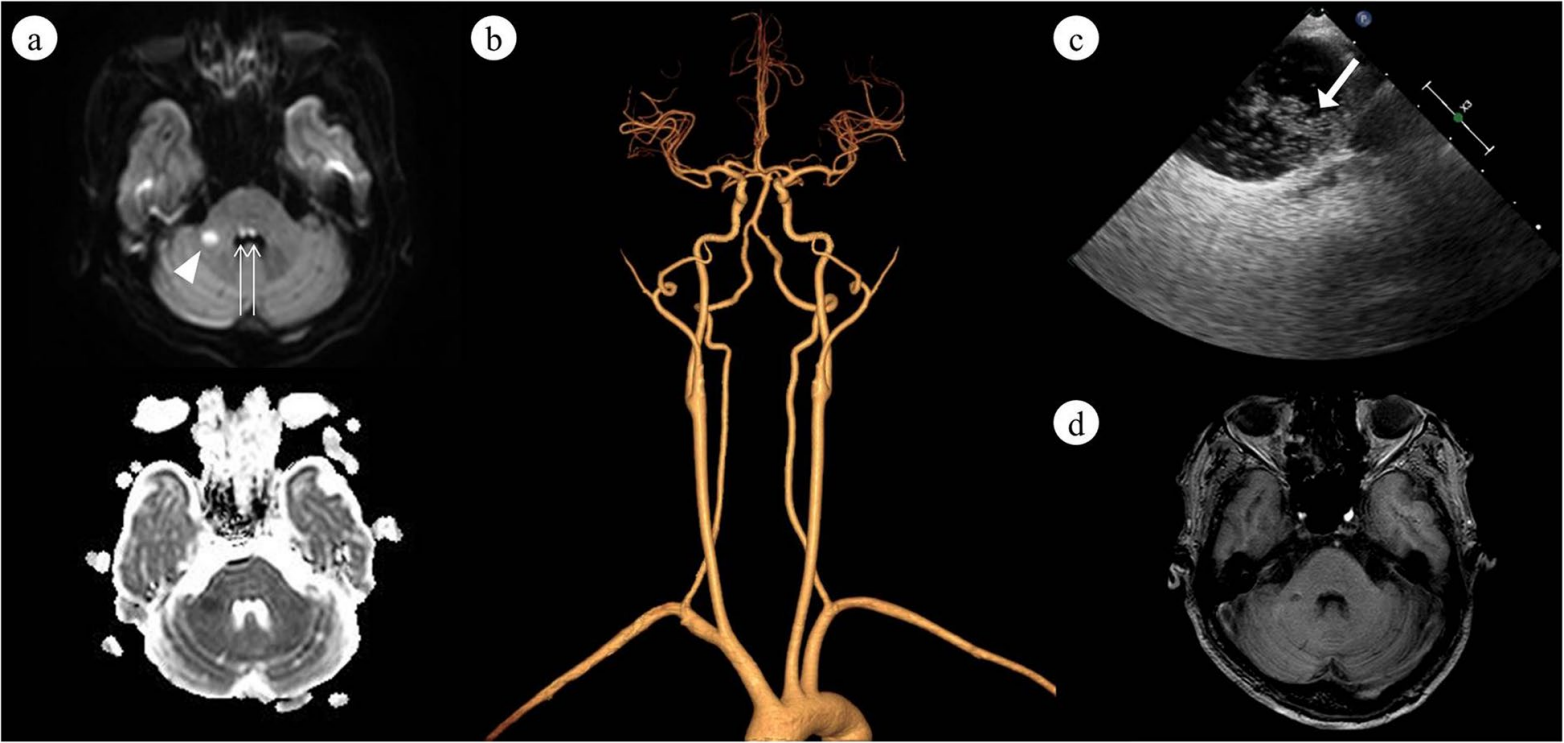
a Diffusion-weighted image 1 day after disease onset revealed increased restricted diffusion signal in the right middle cerebellar peduncle (arrowhead) and bilateral dorsal pontine tegmentum (thin arrows), the latter of which was consistent with the involvement of facial colliculus. The lesions showed correspondent hyposignal on attenuated diffusion coefficient map. b CT angiography of head and neck did not show obvious stenosis in the anterior and posterior circulation. c Right-to-left mircobubbles (thick arrow) were observed on contrast-enhanced transoesophageal echocardiography during Valsalva maneuver. d Follow-up MRI at 6 month showed hypointenstiy in the corresponding region on T1 weighted image, indicating old infarction
